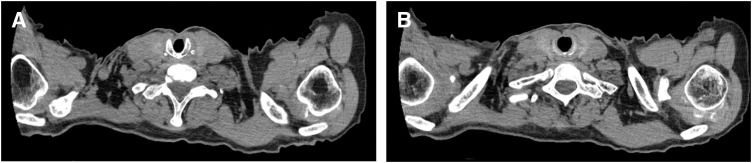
CT performed 120 days after cricotracheostomy. (A) The anterior portion of the cricoid cartilage was removed, and the absence of granulation formation and stenosis was confirmed at the removed portion. (B) The absence of tracheal stenosis was also confirmed below the 1st tracheal cartilage.
